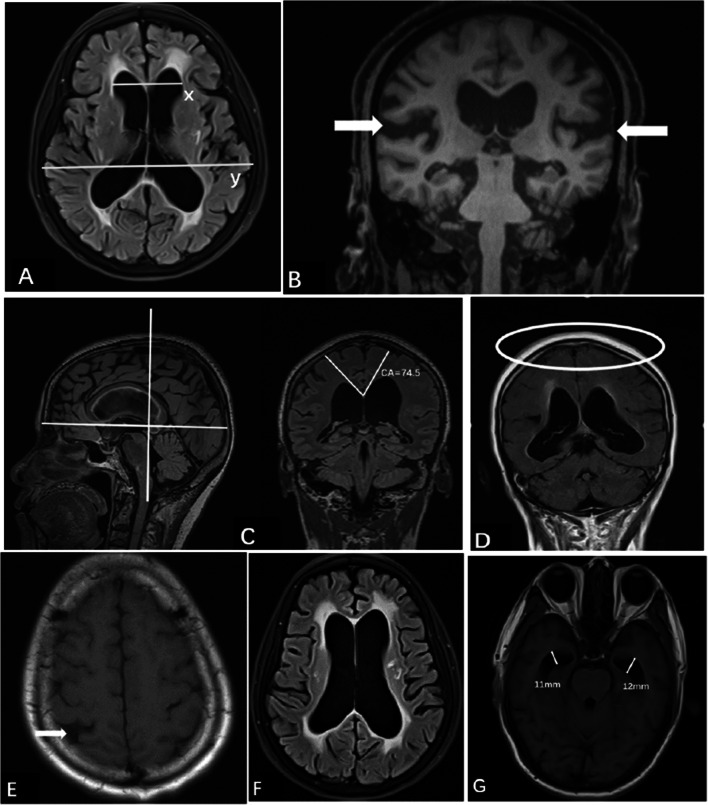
MR images of seven patients with iNPH. A Evans index = A/B. B Enlarged Sylvian fissures. Narrow medial sulci and two focally dilated sulci on the left side. C Callosal angle. D Tight high convexity. E Focally dilated sulci. F PVH graded as 2. G Dilated temporal horns. iNPH, idiopathic normal-pressure hydrocephalus; PVH, periventricular hyperintensities
